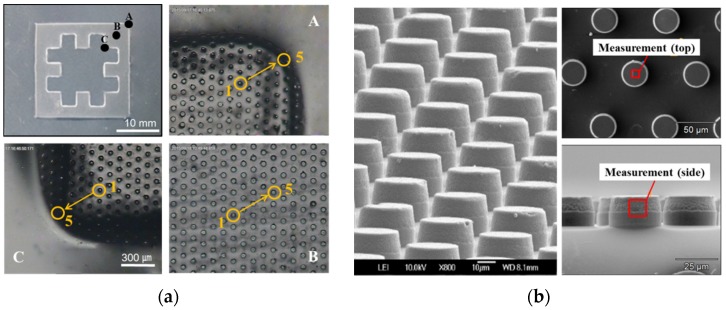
Replicated micropatterns by selective ultrasonic imprinting: (a) replicated micropatterns in different regions; and (b) SEM images of the replicated micropillars in Region B.
